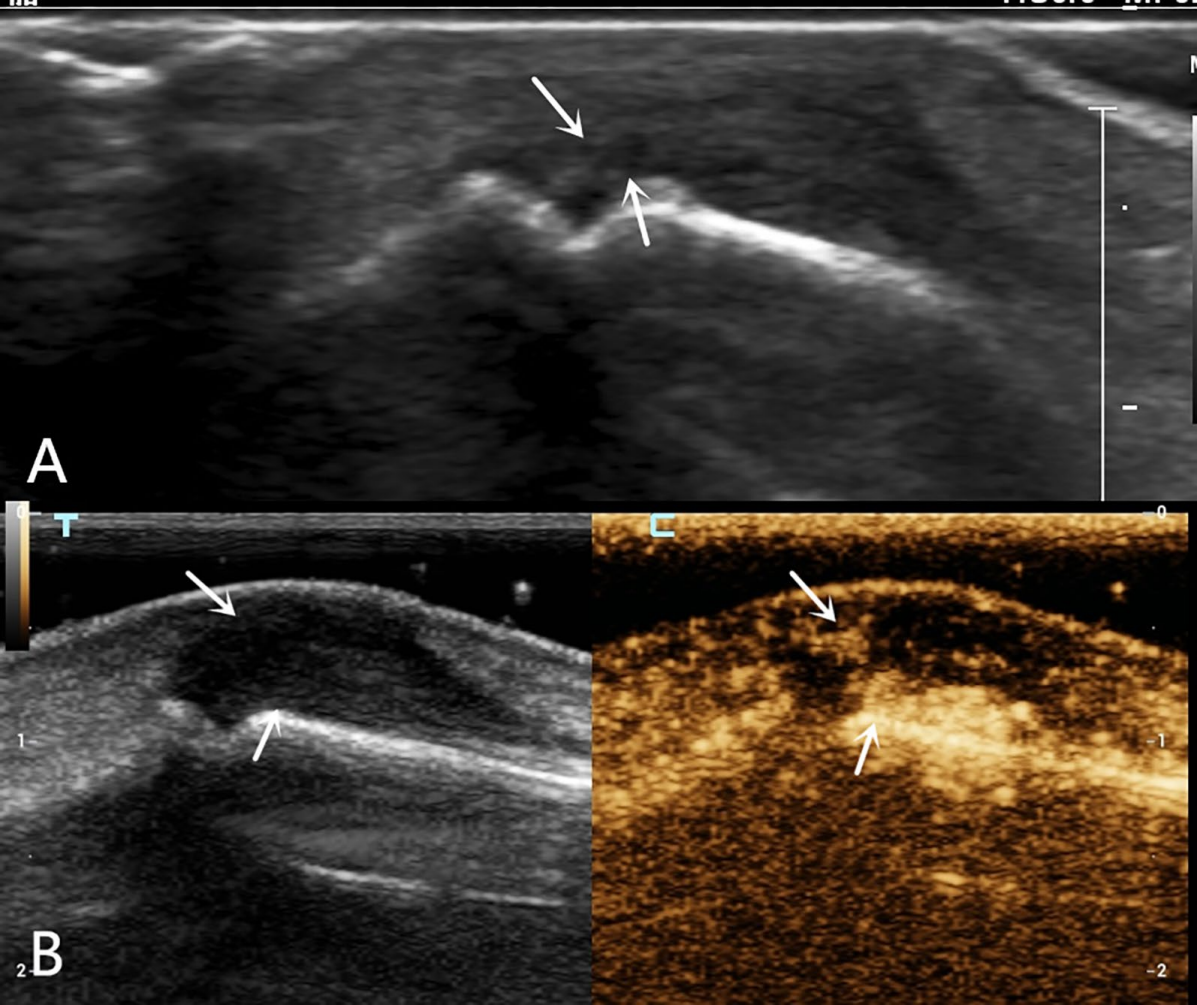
Ultrasound examination of a 53-year-old female with a second left finger tendon injury. A. Two-dimensional ultrasound shows thickening and reduced echogenicity of the extensor tendon at the level of the metacarpophalangeal joint, with suspicious fissure-like hypoechogenicity within the tendon (indicated by a white arrow). B. CEUS shows “triangular” filling perfusion in the superficial and deep layers of the tendon (indicated by a white arrow)
